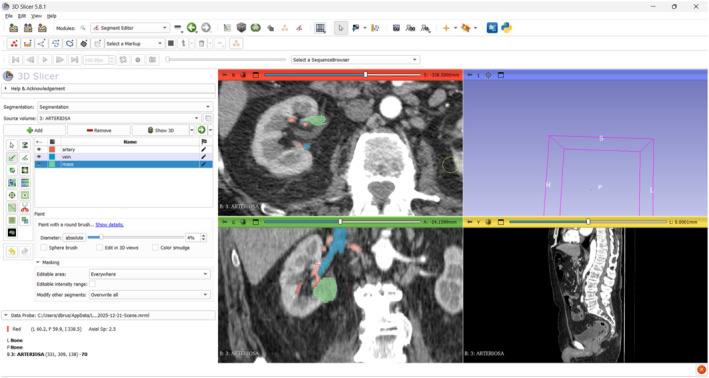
Manual ground‐truth segmentation performed in 3D Slicer (v5.8.1). Axial and coronal CT slices show expert annotation of renal artery (red), renal vein (blue), and renal mass (green). Only vascular segmentations were included in the training dataset; tumour delineation was generated for the anatomical context and excluded from model development.
